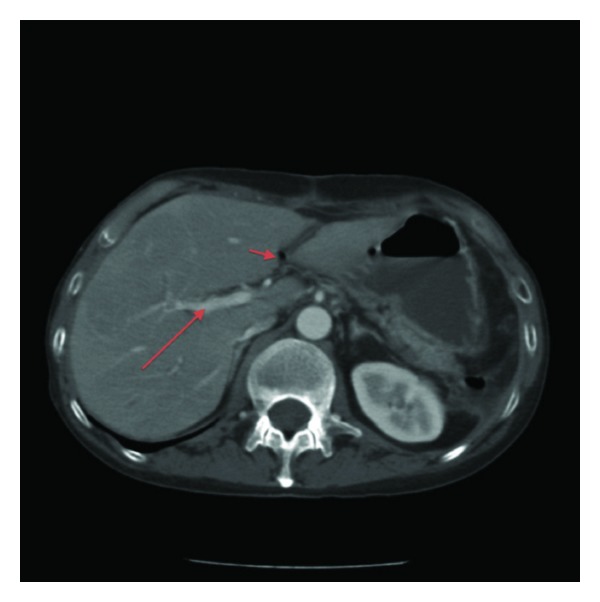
CT scan showing gas in portal vein and a liver abscess.
